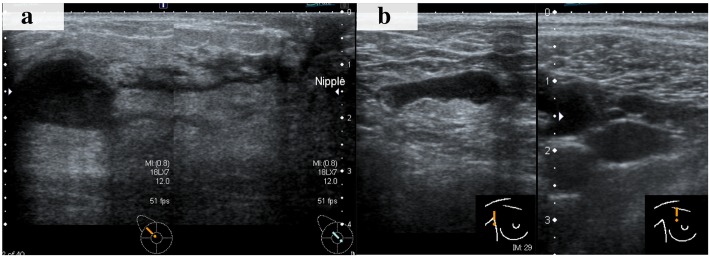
Ultrasound (US) showed an 18 × 16-mm, irregular-shaped, and hypoechoic mass with a suspicion of a spread to the nipple inside the duct (a). One of the swollen lymph nodes in her right axilla (b)
